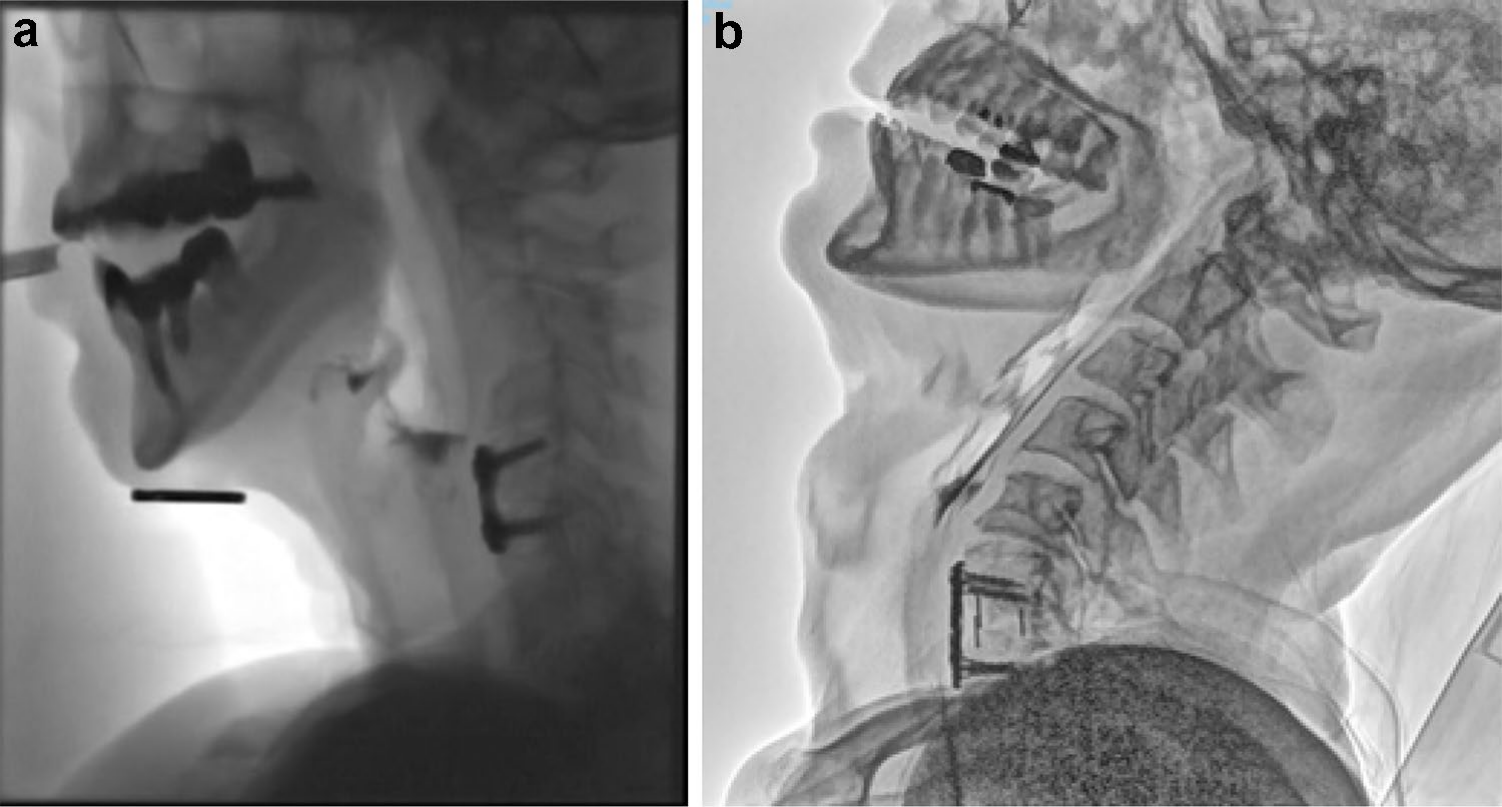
a Radiographic image of a C4-5 plate with posterior pharyngeal wall thickening (3 weeks post surgery). b Radiographic image of C6/7 anterior fixation with plate with altered C-spine alignment (5 months post surgery) (with permissions)
